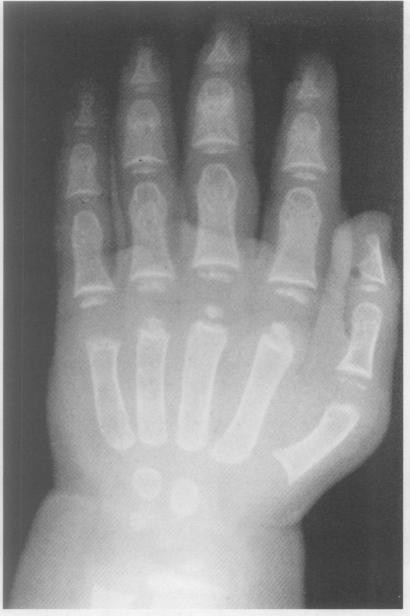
A wrist/hand X-ray of a female with MSS, at chronological age of 18 weeks displaying wide phalanges & stippled epiphyses, showing bone age of 1.9 years [9].
